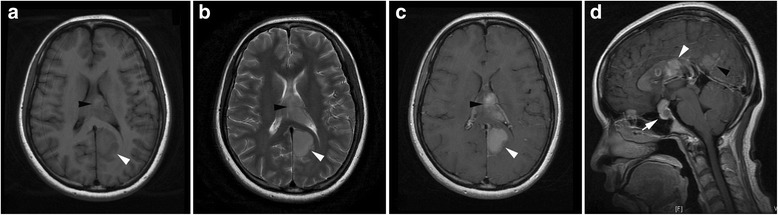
Axial T1 and T2-weighted images showed mass lesions in callosum (*black arrowhead*), left occipital lobe (*white arrowhead*), saddle area and anterior skull base (*white arrow*) respectively, which displayed enhancement in enhanced axial T1 and sigttal T1-weighted images. a Axial T1WI. b Axial T2WI. c Enhanced axial T1WI. d Enhanced sigttal T1WI
